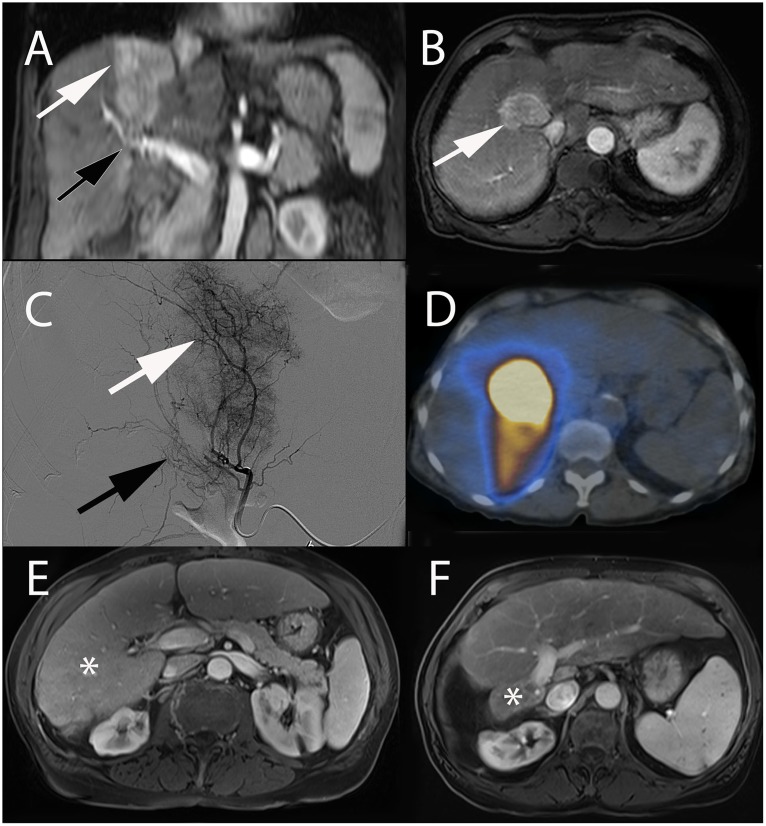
Radiation ablative extended right hepatic lobectomy with SIRT in a 65-year-old female patient with hepatitis C cirrhosis and HCC. Coronal (A) and axial (B) contrast-enhanced (arterial phase) T1-weighted MR images showing large area of enhancement corresponding to HCC centered in segment VIII and IV (white arrow). Note the tumoral portal vein invasion (black arrow). (C) Selective segment VIII angiography demonstrated a large area of contrast blush corresponding to the tumor (white arrow) and portal vein invasion (black arrow). (D) SPECT/CT fused axial image acquired after administration of ^90^Y-microspheres showing activity mostly confined to segment VIII. Axial contrast-enhanced (portal venous phase) T1-weighted MR image obtained before (E) and after (F) two consecutive SIRT. Note post-treatment radiation ablative extended right hepatic lobectomy (^∗^ = segment IV-VIII), with marked compensatory left liver hypertrophy.
